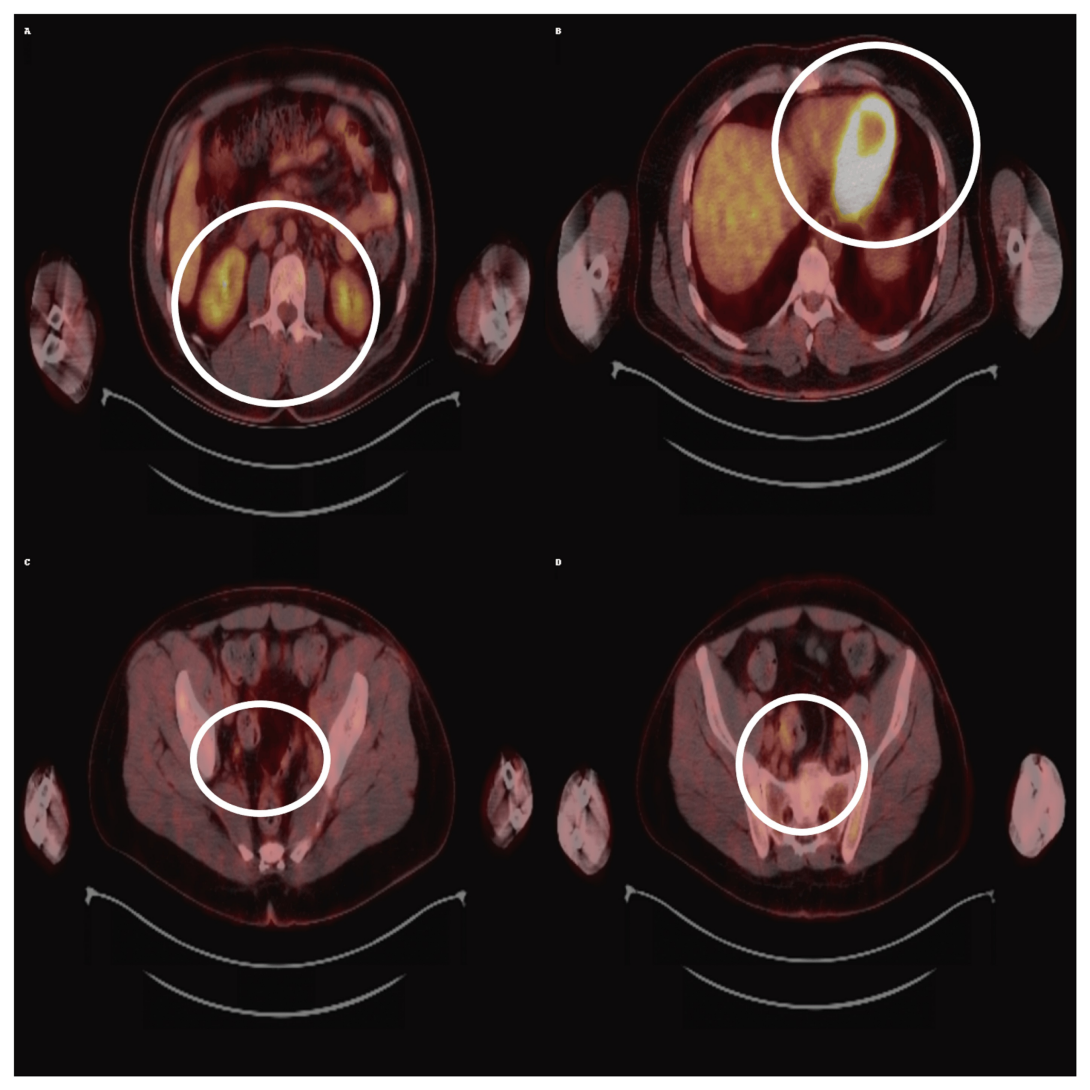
PET/CT scan in April 2025 showing partial metabolic response after systemic therapy. Axial fused PET/CT images (A-D) demonstrate a marked reduction in lesion uptake compared to November 2024, with standardized uptake value (SUVmax) decreasing to 1.78. Mild splenic uptake persisted, as indicated by the white ellipses.
